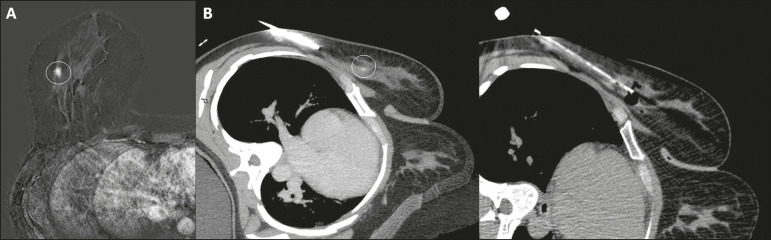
A: MRI showing a mass with irregular margins in the outer quadrants of the right breast (circle). B: CT showing the same mass in the right breast (circle) and needle positioned to perform the biopsy.
